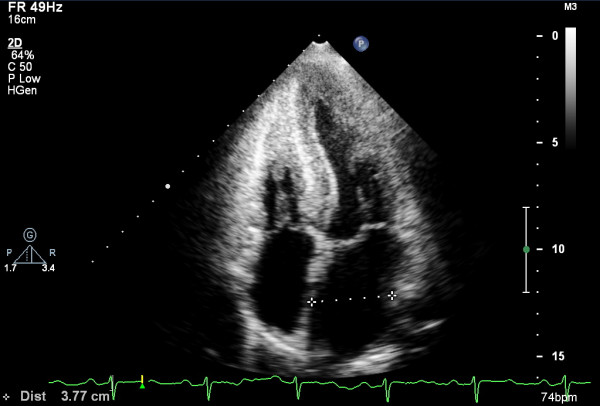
Echocardiographic findings of the patient. Apparent left ventricular hypertrophy and “snowstorm” appearance of the myocardium are suggestive of an infiltrative cardiomyopathy.
